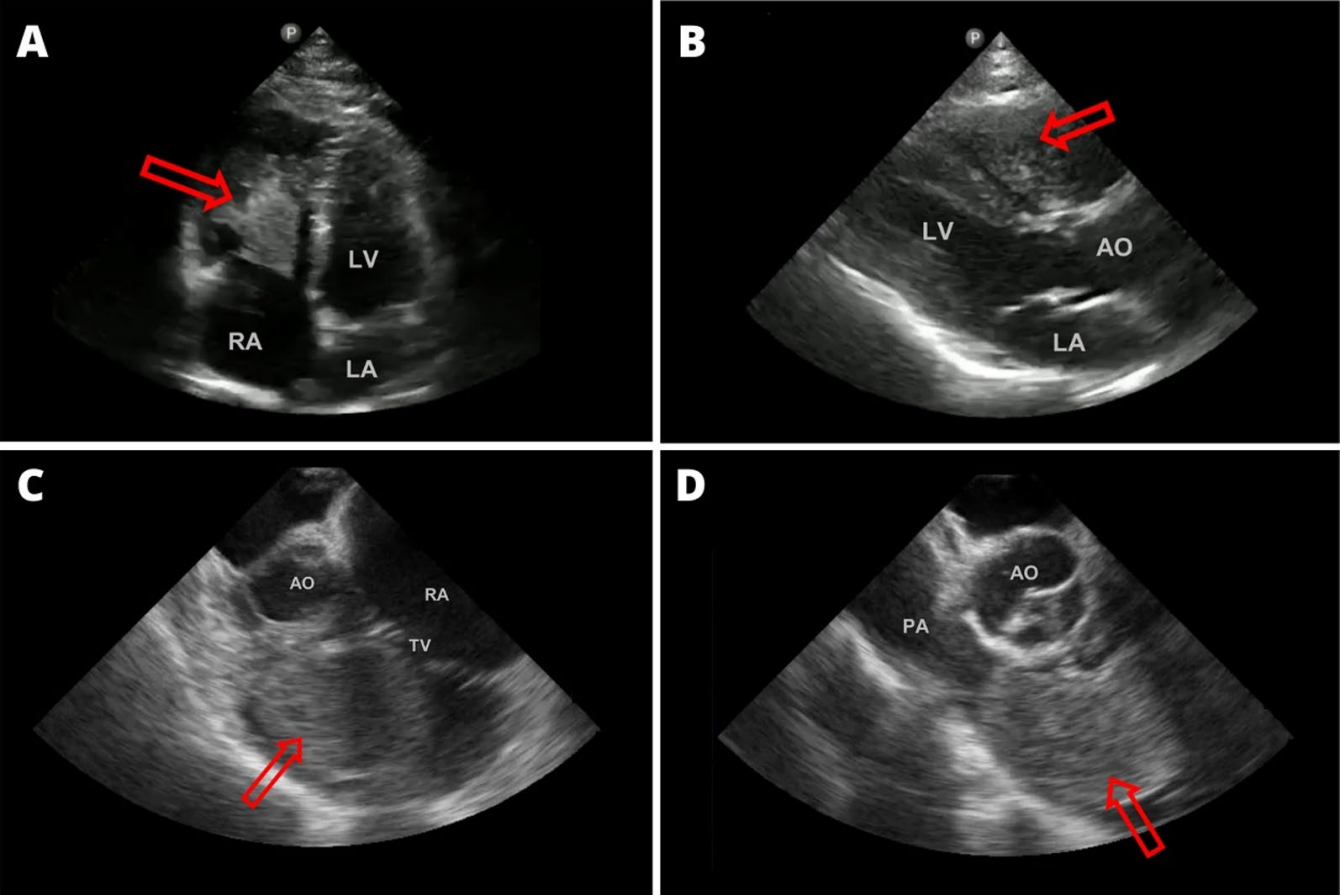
A Four-chamber apical view, transthoracic echocardiography, showing a heterogeneous, pedunculated mass (arrow) protruding toward the septal leaflet of the tricuspid valve. B Long-axis parasternal view, transthoracic echocardiography, displaying a heterogeneous mass (arrow) attached to the interventricular septum. C Mid-esophageal projection, displaying a solid tumor mass protruding toward the tricuspid valve. D Mid-esophageal projection at the level of the great vessels, displaying the tumor mass in the right ventricular outflow tract. RA: right atrium, LA: left atrium, LV: left ventricle, AO: aorta, TV: tricuspid valve, and PA: pulmonary artery
